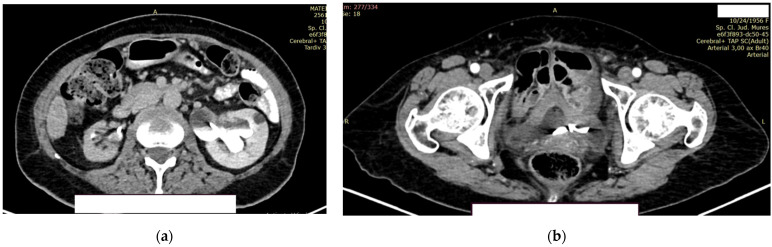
Contrast CT aspect. (a) The right kidney is hypotrophic, with a ureteral stent in place, no stasis, and secretion present. The left kidney also has a ureteral stent, with grade II/III hydronephrosis, and both secretion and excretion are present. (b) The walls of the urinary bladder are concentrically thickened. It is unclear whether the distal intravesical portion of the ureter has any tumor formation.
